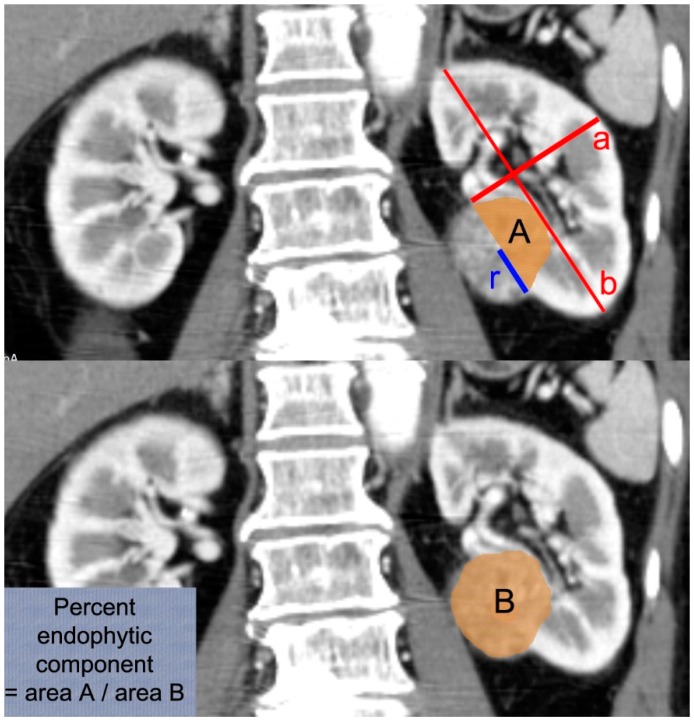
Measurements of the study. Kidney volume was estimated as cylindrical volume using the short (a) and long diameter (b) of the kidney on the three-dimensional computerized tomography (CT) image. The tumor volume was estimated as a ball with its radius of maximal tumor radius (r). Percent endophytic component was measured as a percentage ratio of endophytic tumor area (A) to whole tumor area (B) on the CT image where the maximal tumor area was observed.
